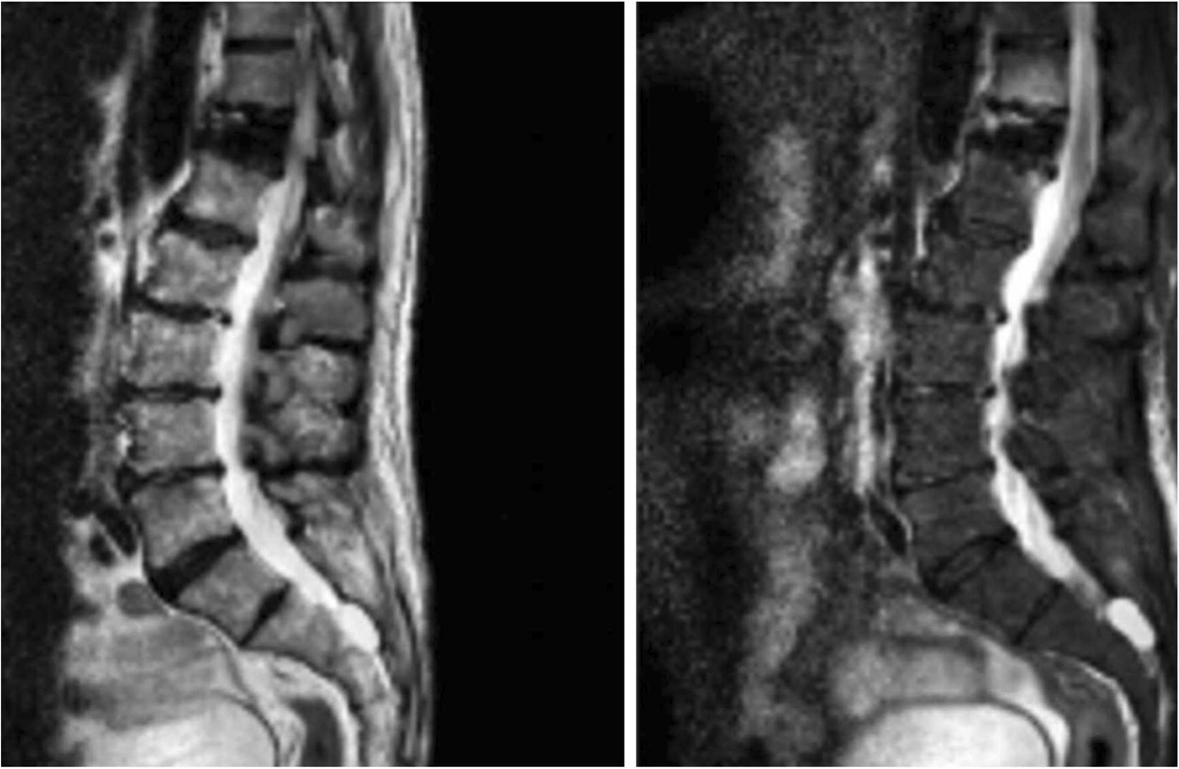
T2-weighted magnetic resonance imaging scans and short tau inversion recovery sequence sagittal cuts showing wedge fracture of T12.
